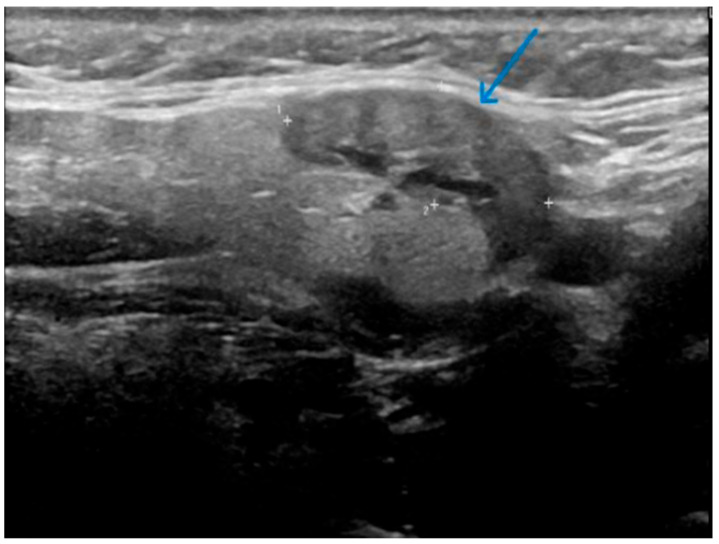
Patient X—Initial ultrasound scan of affected SMG. Well-defined, focal hypoechoic area (indicated by the blue arrow) in the left SMG with mild focal duct dilatation. The "+" markers represent measurement callipers to denote the borders of the hypoechoic lesion.
